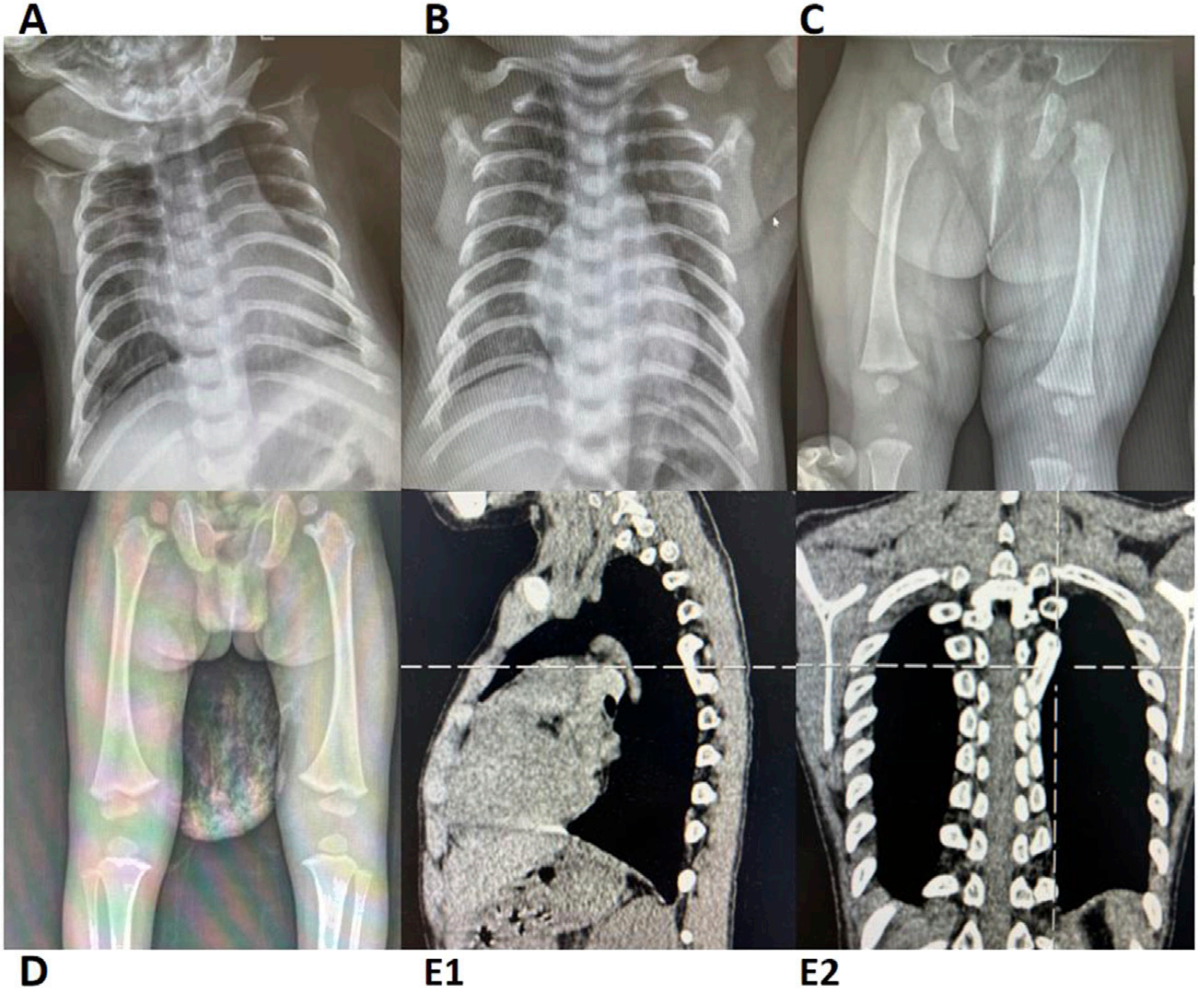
Radiographic and CT Imaging of Skeletal Abnormalities in Cases 2, 4, 5, 15, and 17 (A) Case 2; (B) Case 4; (C) Case 15; (D) Case 17; (E1-2) Case 5. Cases 2 and 4: Chest X-rays reveal a barrel-shaped thorax with bilateral rib enlargement at the anterior ends. Cases 15 and 17: Lower limb X-rays show bilateral femoral head epiphyseal dysplasia. Case 5: Chest CT demonstrates a bony bridge connecting the posterior ends of the left 5th and 6th ribs. CT, computed tomography.
